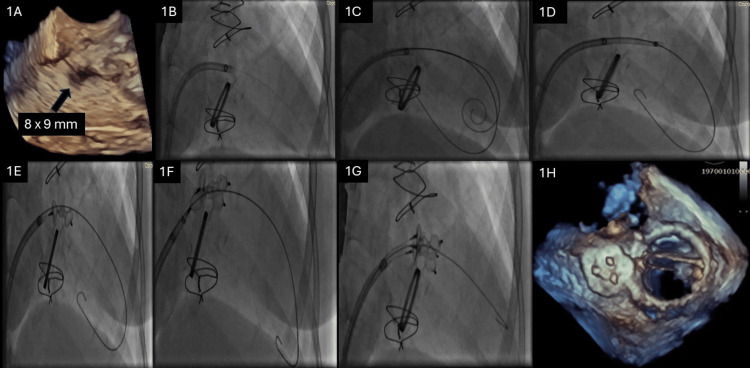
Three-plug technique for mitral paravalvular leak closure in Marfan syndrome (A) Pre-procedural 3D echocardiography with 8 x 9 mm defect. (B) Defect crossed with 0.014-inch wire through 15F SGC. (C) Super stiff wire and 0.035-inch buddy-safety wire through the defect. (D) A 7F guide sheath insertion. (E) First AVP III 14 x 5 mm deployment. (F) Second AVP III 14 x 5 mm deployment. (G) Third AVP III 10 x 5 mm deployment. (H) Final result. 3D, three-dimensional; SGC, steerable guide catheter; AVP, Amplatzer Vascular Plug
